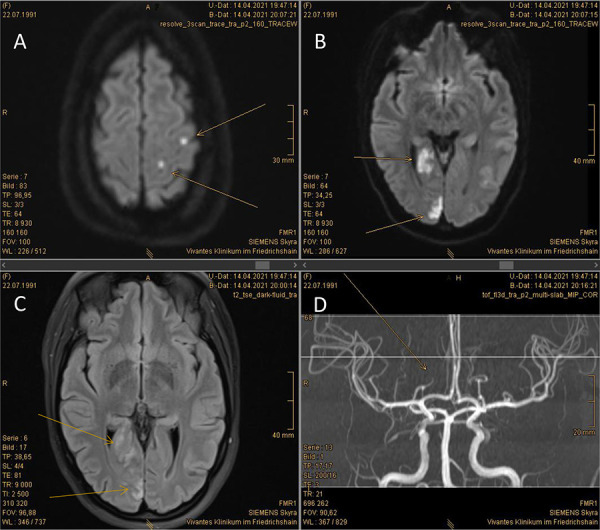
MRI scan of the brain owing to left homonymous hemianopia. A: Diffusion-weighted magnetic resonance imaging (DWI) showing at least two acute non-embolic microangiopathic infarctions of the left cerebral hemisphere (arrows). B: DWI sequence showing an acute territorial infarction of the right posterior cerebral artery (arrows). C: FLAIR sequence of the brain confirming right-sided posterior infarction (arrows). D: MR angiography of the intracranial arteries showing a peripheral thrombosis of the right posterior cerebral artery (arrow).
